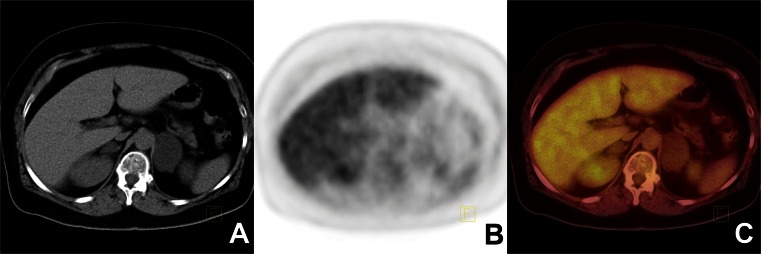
^18^F-FDG PET/CT findings in a 55-year-old women with disseminated metastasis from an unknown primary cancer (a CT image, b PET image, c fused PET/CT image). The ^18^F-FDG PET/CT image shows low ^18^F-FDG uptake. The SUVmax of the enlarged right adrenal tumour was 2.3 and the attenuation value −11 HU; the liver SUVmax was 4. These findings were consistent with a lipid-rich adenoma
